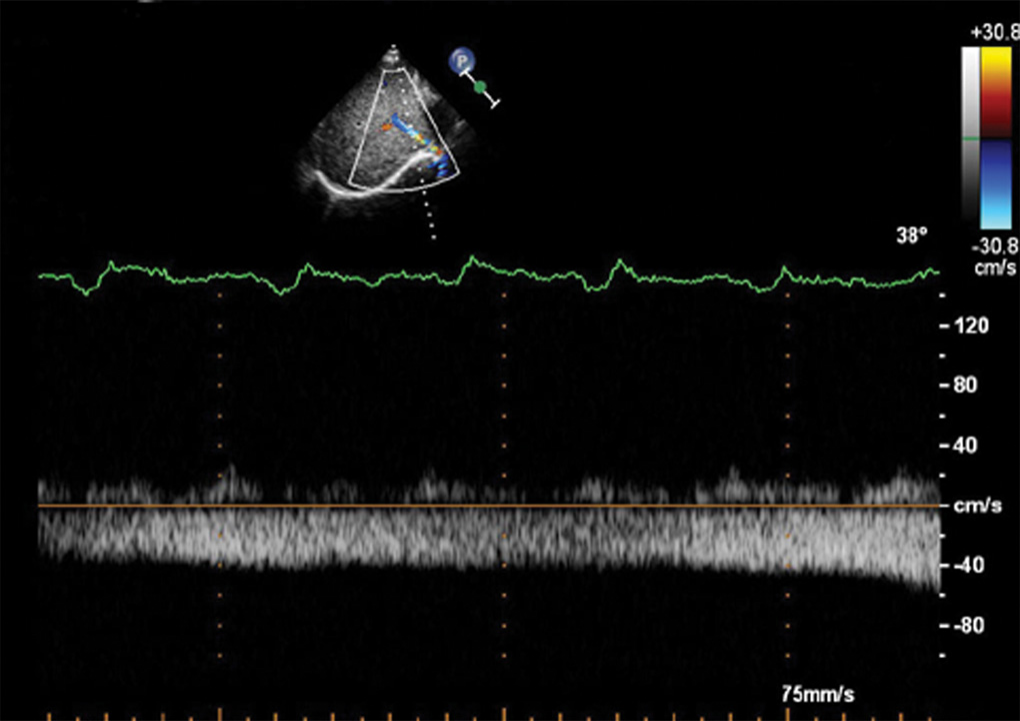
Mono-phasic Doppler flow spectra of the MHV in the PH group. Doppler spectra of MHV obtained from a patient with atrial septal defect with the MPAP of 33 mmHg. The waveform of MHV is flat and mono-phasic.
